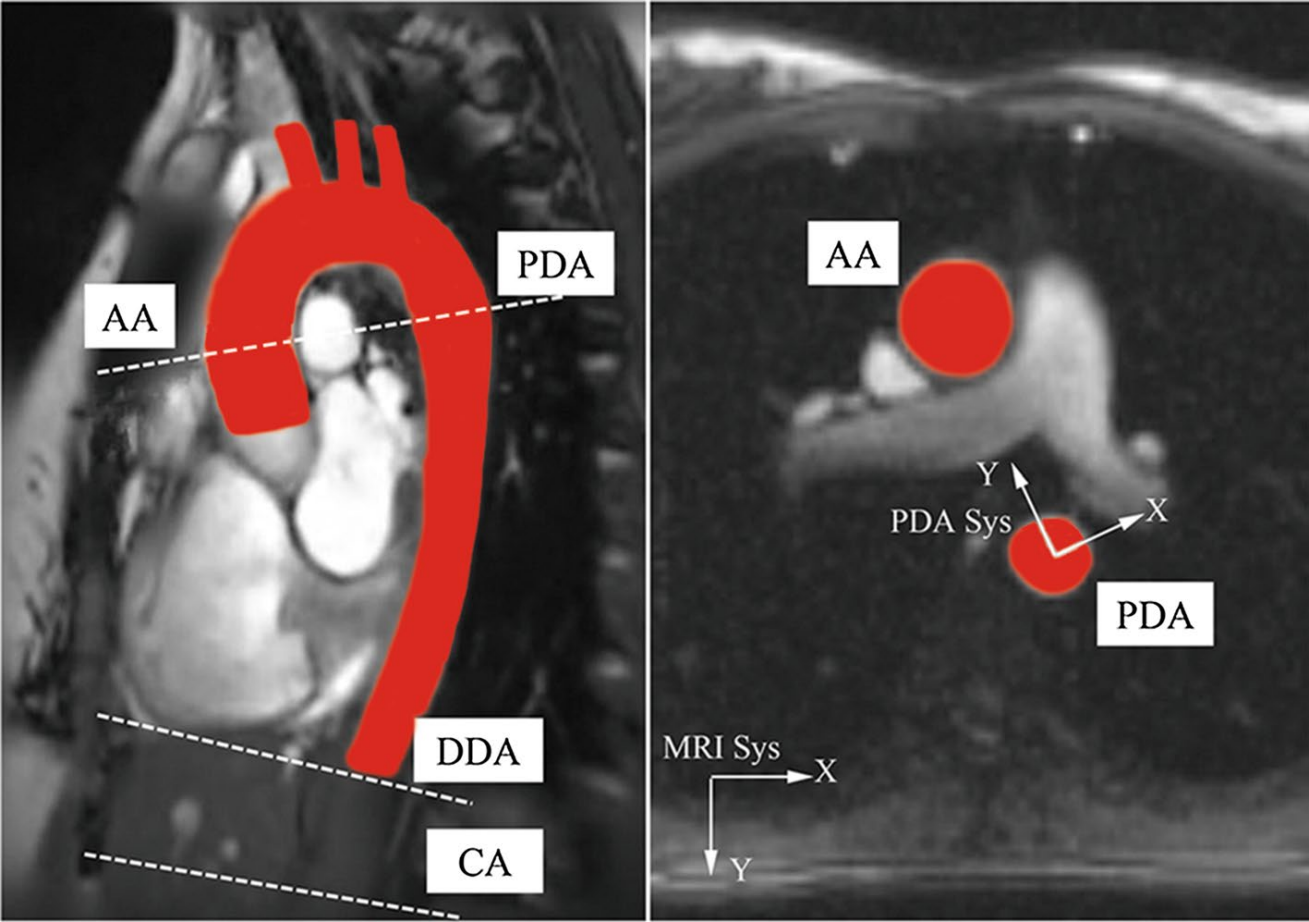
Oblique sagittal and AA-perpendicular MRI images. The region in red colour corresponds to the aorta. Left: oblique sagittal MRI image showing the locations of different aortic sections. Right: the MRI image perpendicular to the AA for measuring its in-plane motion. MRI Sys and PDA Sys are the abbreviations for MRI reference system and PDA system respectively
